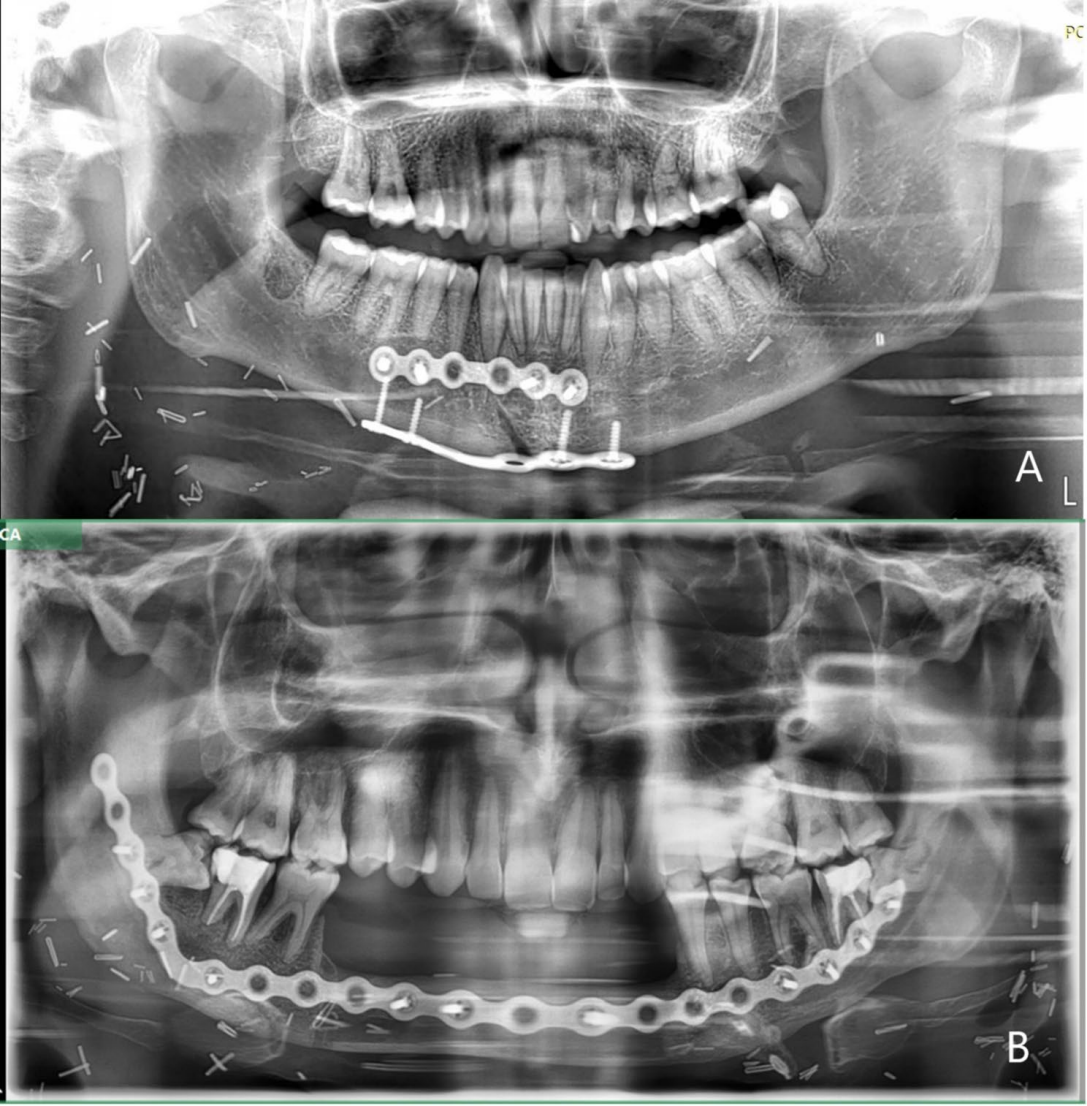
Panoramic radiographs of patients who underwent right paramedian mandibulotomy (tongue SCC) (A), and left and right segmental mandibulectomy (Floor of the mouth and tongue SCC) (B)
